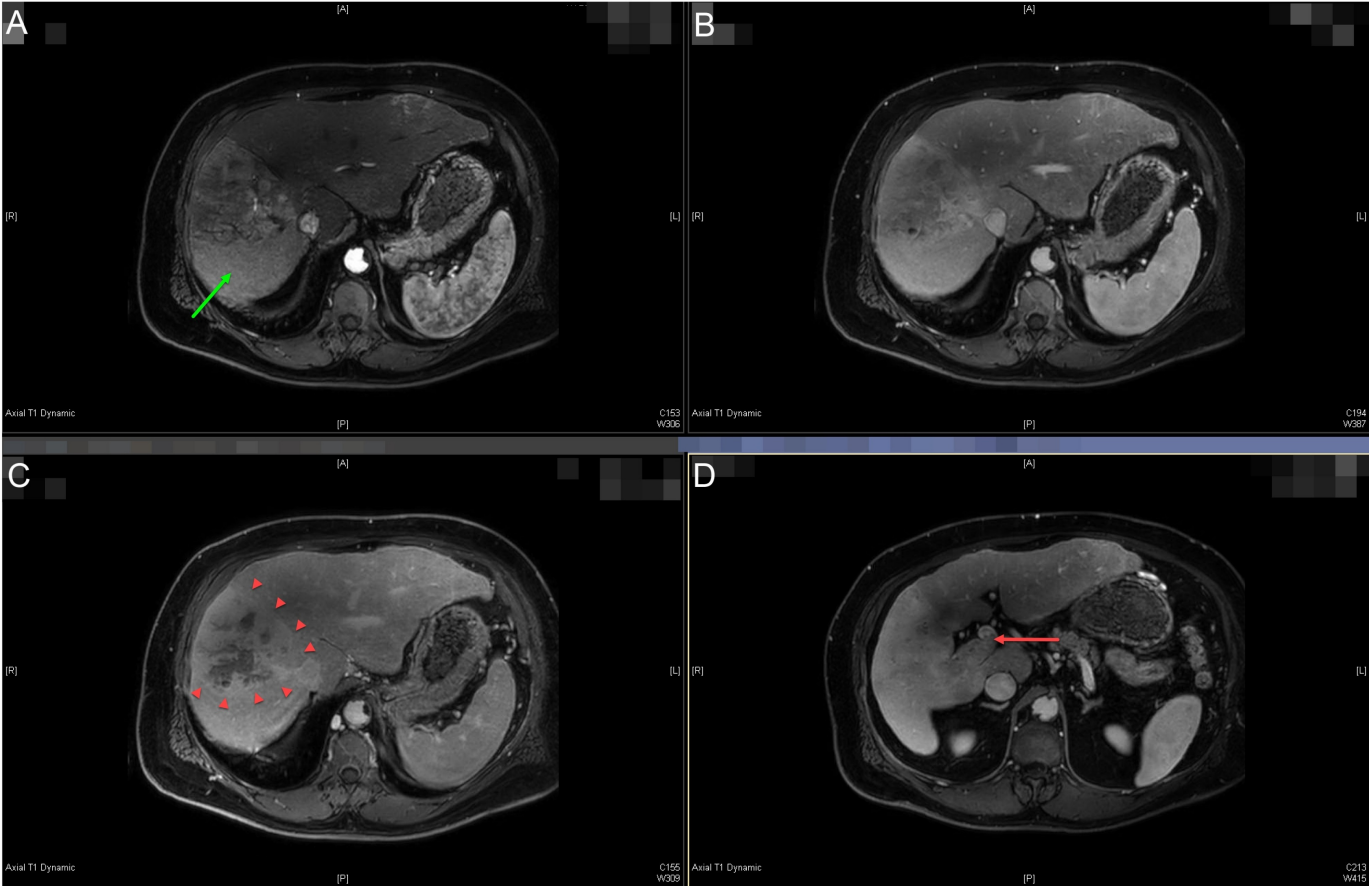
Pre-treatment diagnostic magnetic resonance imaging (MRI) of the abdominal scan. Multi-phase liver protocol MRI abdomen axial images showing arterial (A), venous (B), and delayed (C) phases of the diffusely infiltrative and heterogeneously enhancing hepatocellular carcinoma tumor involving segments five through eight (best seen on panel C, arrowheads). The portal vein tumor thrombus is seen extending into the main portal vein on venous delay (D - red arrow). Peri-tumoral perfusion abnormalities are seen in panel A (green arrow).
